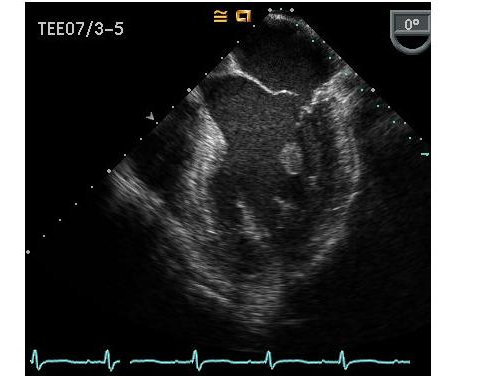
Transesophageal Echocardiogram showing an echo density in the left ventricle suggestive of partial ruptured anterolateral papillary muscle.
